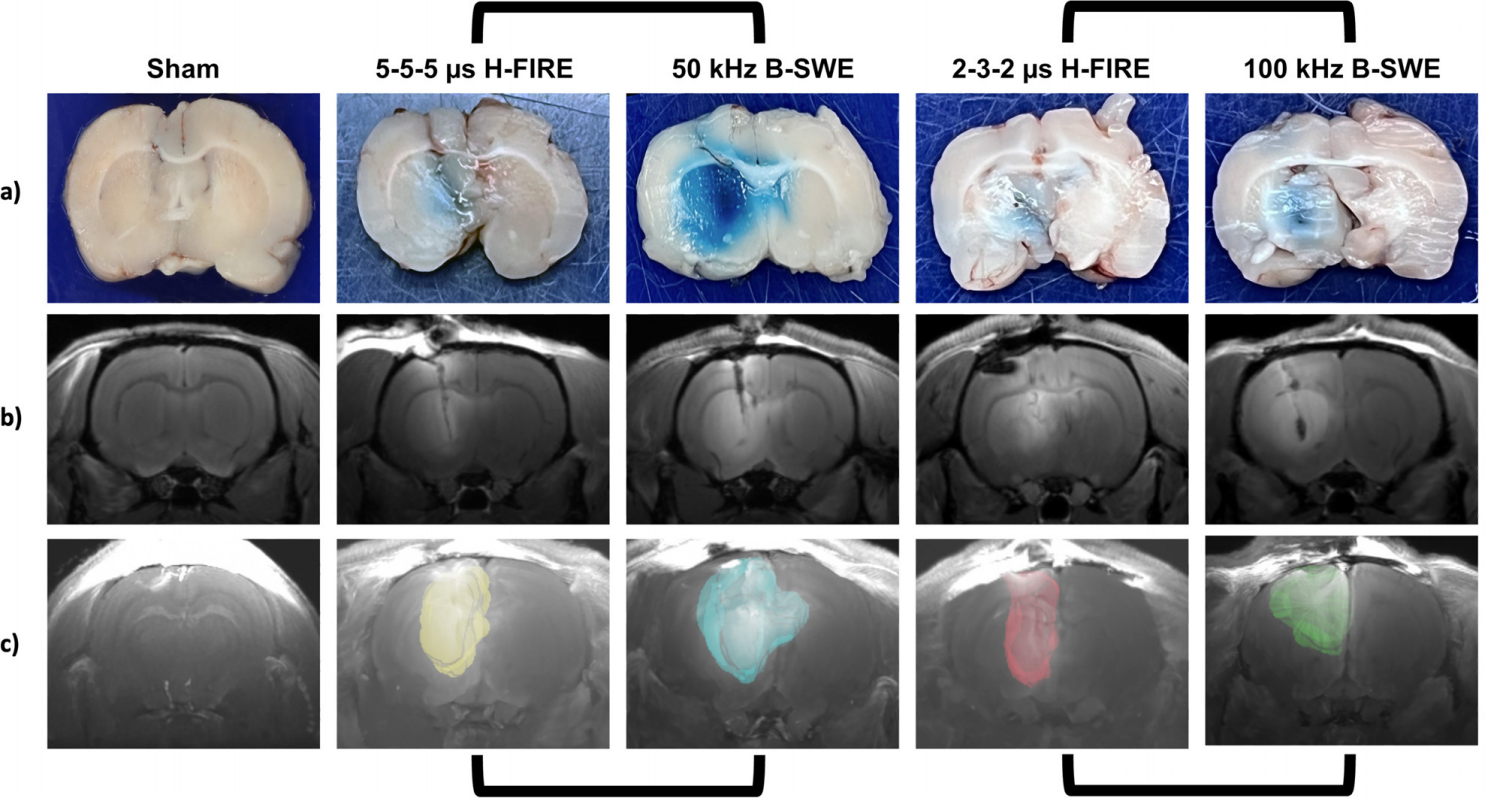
A visualization of BBB disruption 1 h post-treatment as noted by the diffusion of normally impermeant (a) Evans blue dye (EBD) and (b) gadopentetate dimeglumine (Gd-DTPA) into the striatum. Volumetric measurements of EBD were taken by measuring tissue sections while (c) contrast-enhanced regions of Gd-DTPA uptake on T1W MRI scans were contoured and quantified. Both EBD and Gd-DTPA volumetric measurements demonstrate greater BBB disruption from an equivalent B-SWE protocol. All tissue sections and scan slices depict planes of BBB disruption along or orthogonal to the electrode insertion tip. Paired protocols are matched via brackets.
